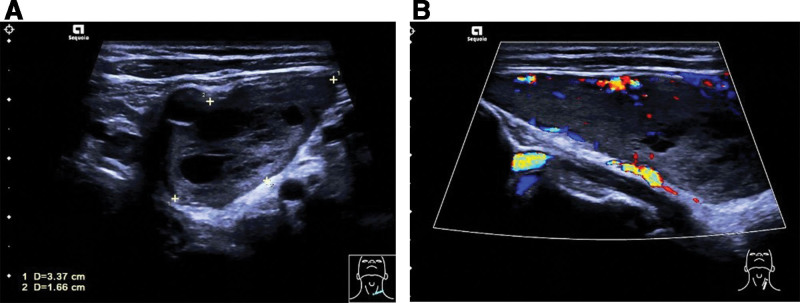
Ultrasound of the neck showing a huge mixed cystic and solid mass (A) in the left supraclavicular region (partially behind the common carotid artery) and vascularity (B).
